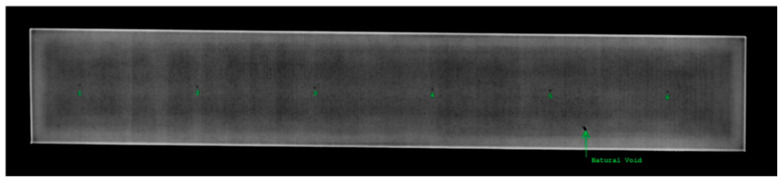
Radiograph of Sample A showing the locations of 6 sub-surface voids.
